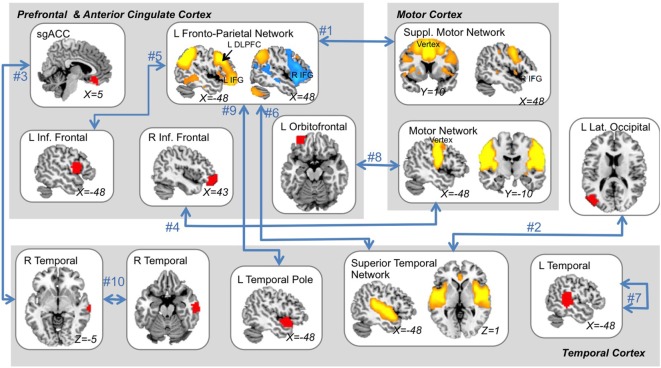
The ten “best” multimodal functional magnetic resonance imaging features. The top 10 features that were most consistently selected across all outer cross-validation splits are displayed. All top features related to functional-connectivity strength among networks (orange and blue regions) and/or single brain areas (red regions) as indicated by blue arrows. A single feature reflected local connectivity strength (i.e., regional homogeneity). Feature rank is displayed next to blue arrows (see also Table 2) and MNI coordinates are given for each slice displayed. L, left; R, right; ACC, anterior cingulate cortex; DLPFC, dorsolateral prefrontal cortex; suppl., supplementary; Inf., inferior.
